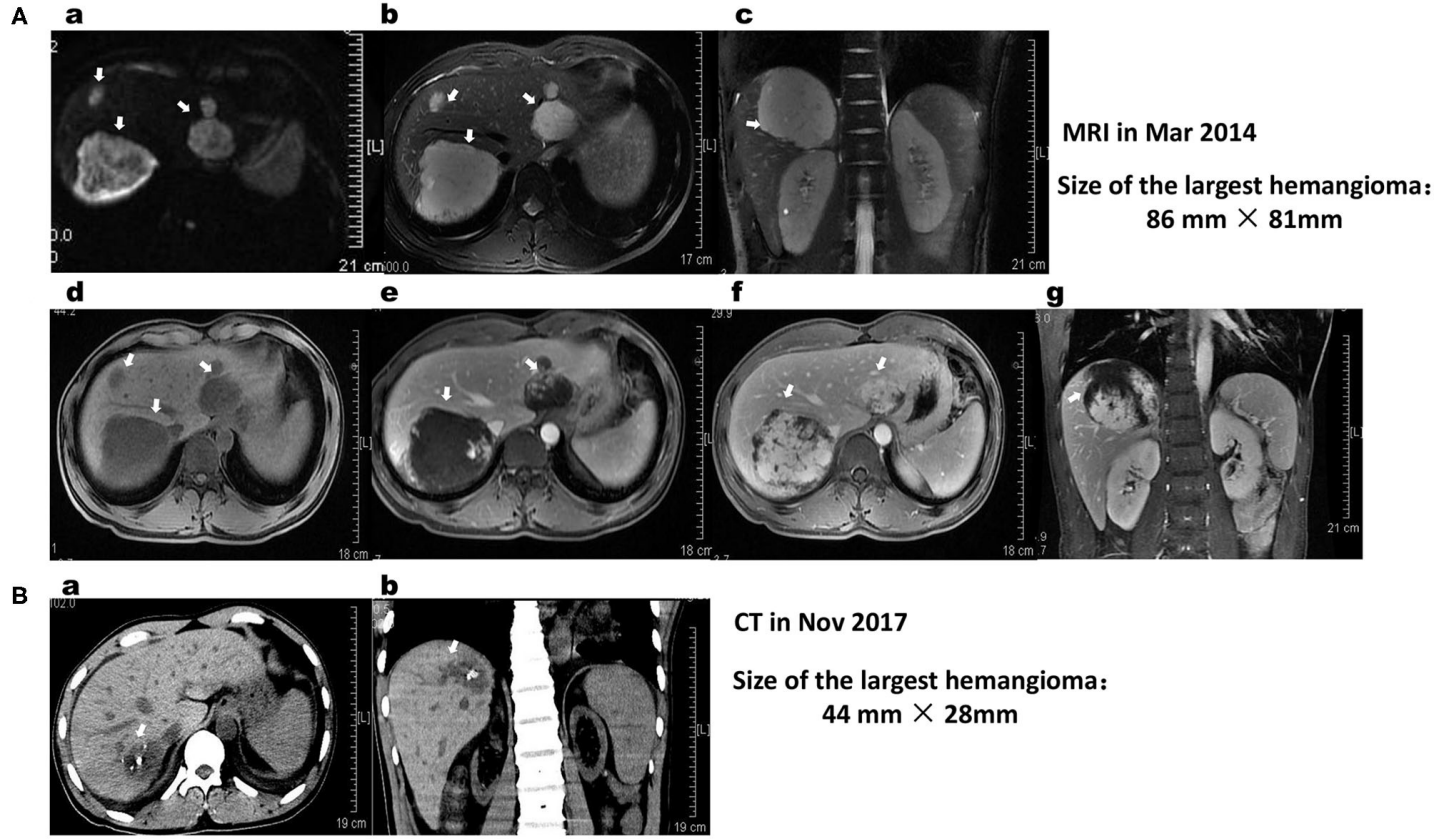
(A) MRI of the liver shows lobulated lesions in the subcapsular aspect of segments 2, 7, and 8 of the liver (white arrows). The largest lesion is located at segment 7, its size is ~86 × 81 × 81 mm, and it is closely related to the liver segments of the right hepatic vein and IVC. The size of the lesion in S2 is ~54 × 44 × 41 mm. The hemangiomas on MRI appear hyperintense on DWI (a) and T2WI (b,c) and hypointense on T1WI (d–g) sequences. On post-contrast dynamic imaging, the lesion demonstrates discontinuous peripheral nodular enhancement on the arterial phase (e) with incomplete centripetal fill-in on the delayed phase (d,f). The findings are consistent with hepatic hemangiomas. (B) Comparing the cross-sectional CT (a) and coronal (b) reformatted CT images with those of MRI images on March 16, 2014, primary multiple hemangiomas in the liver were significantly shrunk, and their boundaries were not clear. The size of the giant hemangioma in segment 7 of the liver was decreased to 44 × 28 mm, with unclear borders and nodular high-density shadows inside it. The lesions are closely related to the liver segments of the right hepatic vein and IVC.
